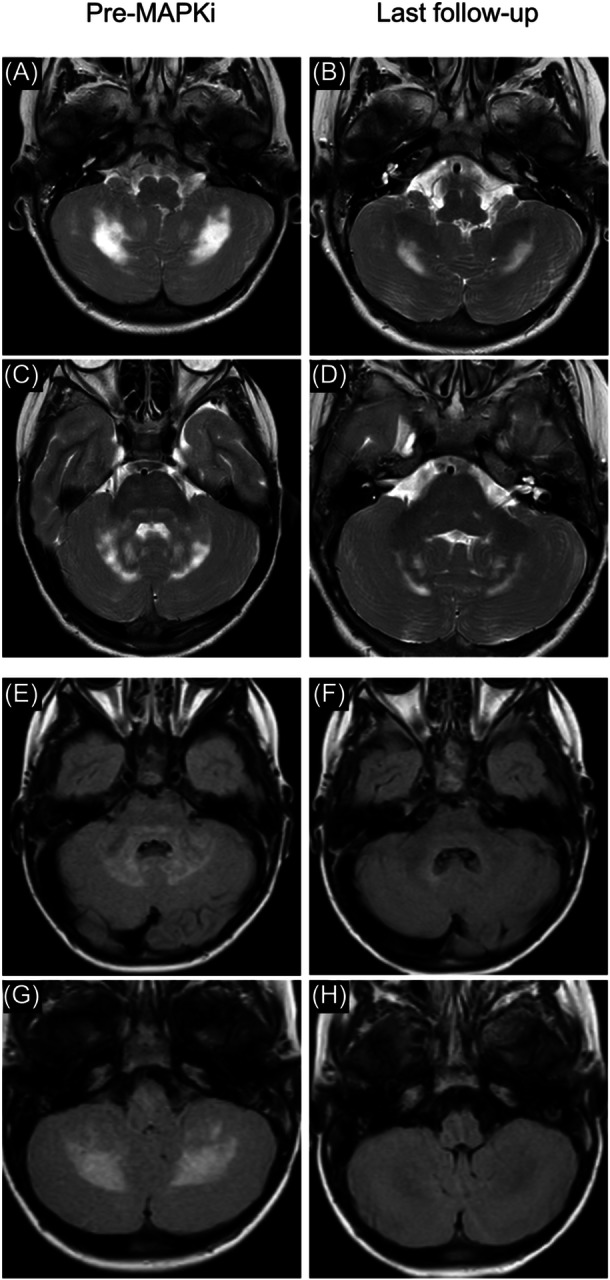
Magnetic resonance imaging response to MAPK inhibitors (MAPKi) of two different patients before treatment initiation, compared with the last follow‐up on treatment. (A–D) Axial T2 images showing areas of hypersignal involving the cerebellar white matter (A), with partial regression of the lesions after MAPKi treatment (B); and areas of hypersignal involving the dentate nuclei, cerebellar white matter, and dorsal pons (C), with complete regression of the lesions in the dorsal pons and partial regression in the dentate nuclei and cerebellar white matter after MAPKi treatment (D). (E–H) Axial FLAIR images showing areas of hypersignal involving the dentate nuclei, the cerebellar white matter, and the dorsal pons (E), with almost complete regression of the lesions after MAPKi treatment (F); and areas of hypersignal involving the cerebellar white matter (G) with complete regression of the lesions after MAPKi treatment (H).
